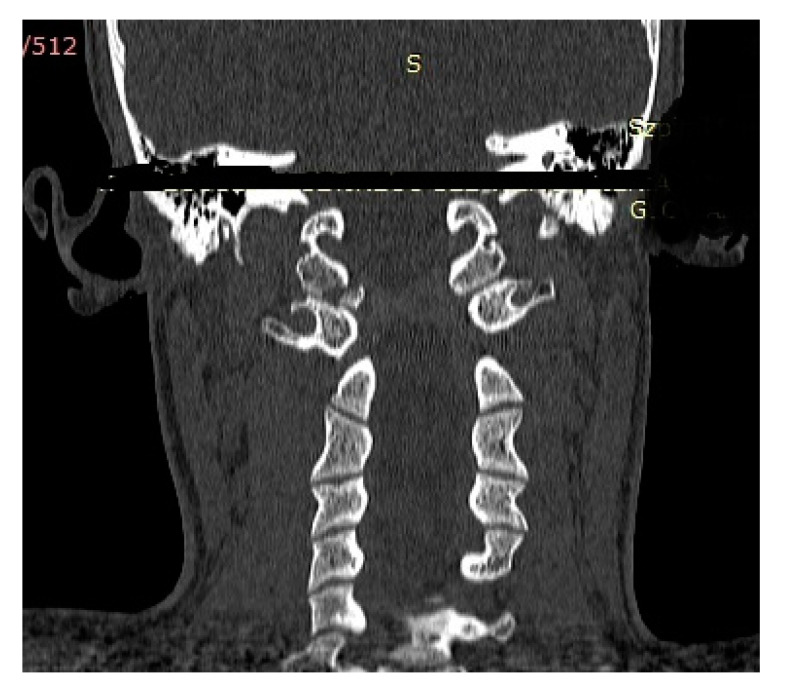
CT scan (frontal plane) of a boy aged 15.2 years (Anderson–Montesano type III, Tuli type IIB injury; patient: P.P.) obtained after admission to the A&E room. Avulsed fragment of right condyle displaced medially by 4.0 mm.
